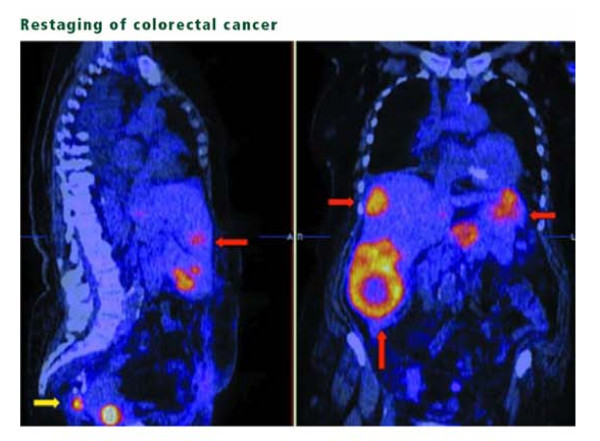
Restaging of colorectal cancer. Sagittal (left) and coronal (right) PET/CT slices of patient with prior surgery and increasing carcinoembryonic antigen show increased FDG uptake in multiple liver lesions(red arrows), as well as recurrence of local disease in the presacral space (yellow arrow).
